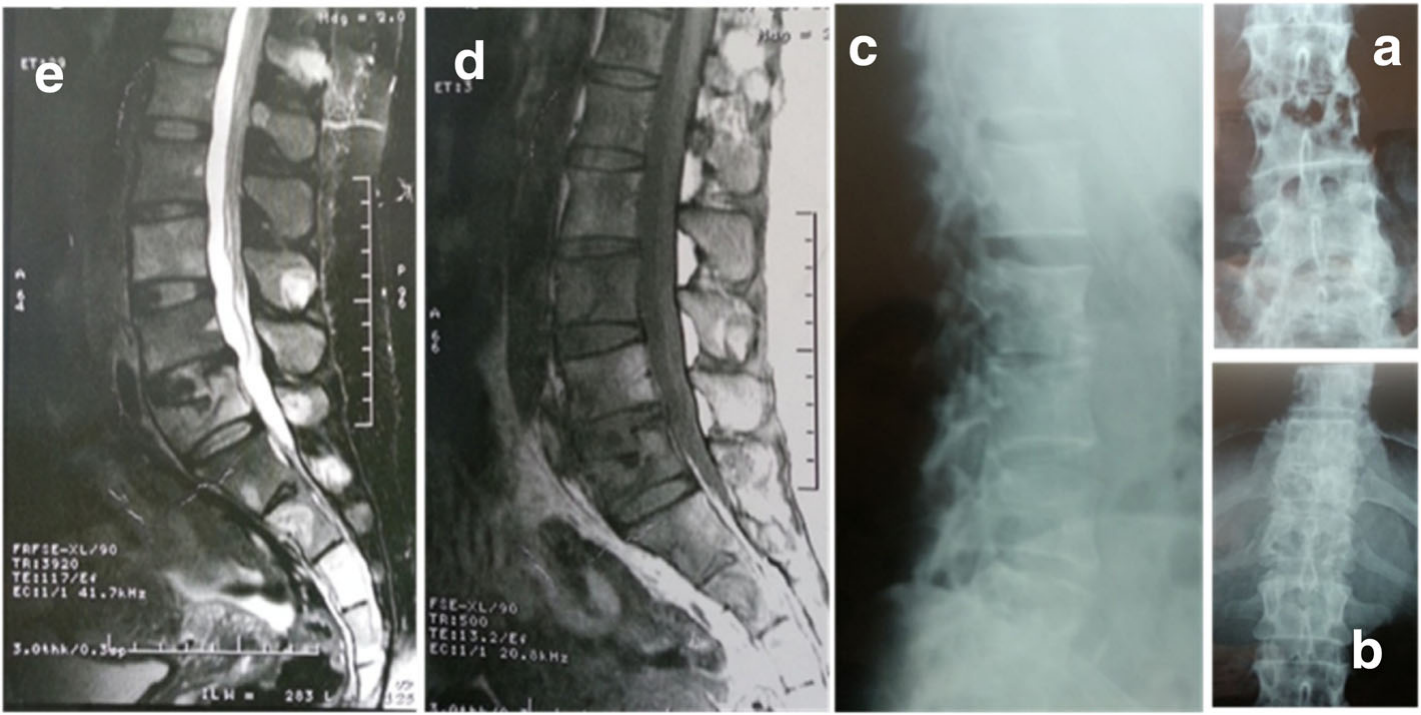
Right*: radiographs of the lumbar spine taking the last dorsal vertebra showing spinal fractures and disc space narrowing. a X-ray of the lumbar spine to face centered on L2 to L3. b X-ray of the lumbar spine to face centered on D10 to D11. c X-ray of the lumbar spine profile centered on L2 to L3. *Left*: the old lumbar MRI showing L5 fracture with abnormal signal de D11 and D12. d T1 sagittal section of lumbar MRI showing L5 fracture. e T2 sagittal section of lumbar MRI showing L5 fracture
